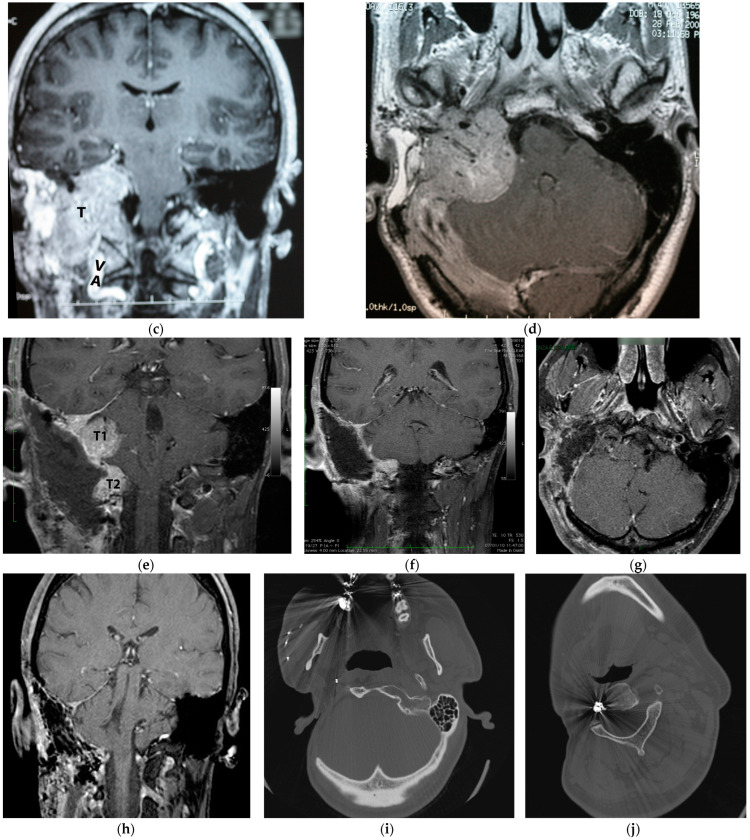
(Case 6) (a,b) Right-sided tympanojugular PGL (C4Di2Vi). (c) Coronal Gd-enhanced T1-weighted magnetic resonance imaging (MRI) shows a large residual tumor extending to the intradural space involving the vertebral artery. (d) Axial Gd-enhanced T1-weighted MRI shows a large residual tumor extending to the intradural space, involving transverse sinus ipsilaterally up to the torcula. (e) Coronal Gd-enhanced T1-weighted MRI after first-stage tumor removal. Note the residual tumor: T1 is in the cerebellopontine angle, and T2 is in the foramen magnum. (f) Coronal Gd-enhanced T1-weighted MRI after second-stage tumor removal, which shows a small residual tumor at the foramen magnum. (g,h) Axial and coronal Gd-enhanced T1-weighted MRI after third-stage tumor removal, which revealed total tumor removal at the level of the foramen magnum. (i,j) Axial CT scan after the third stage shows a coil at the internal carotid and vertebral arteries that are used for artery occlusion.
